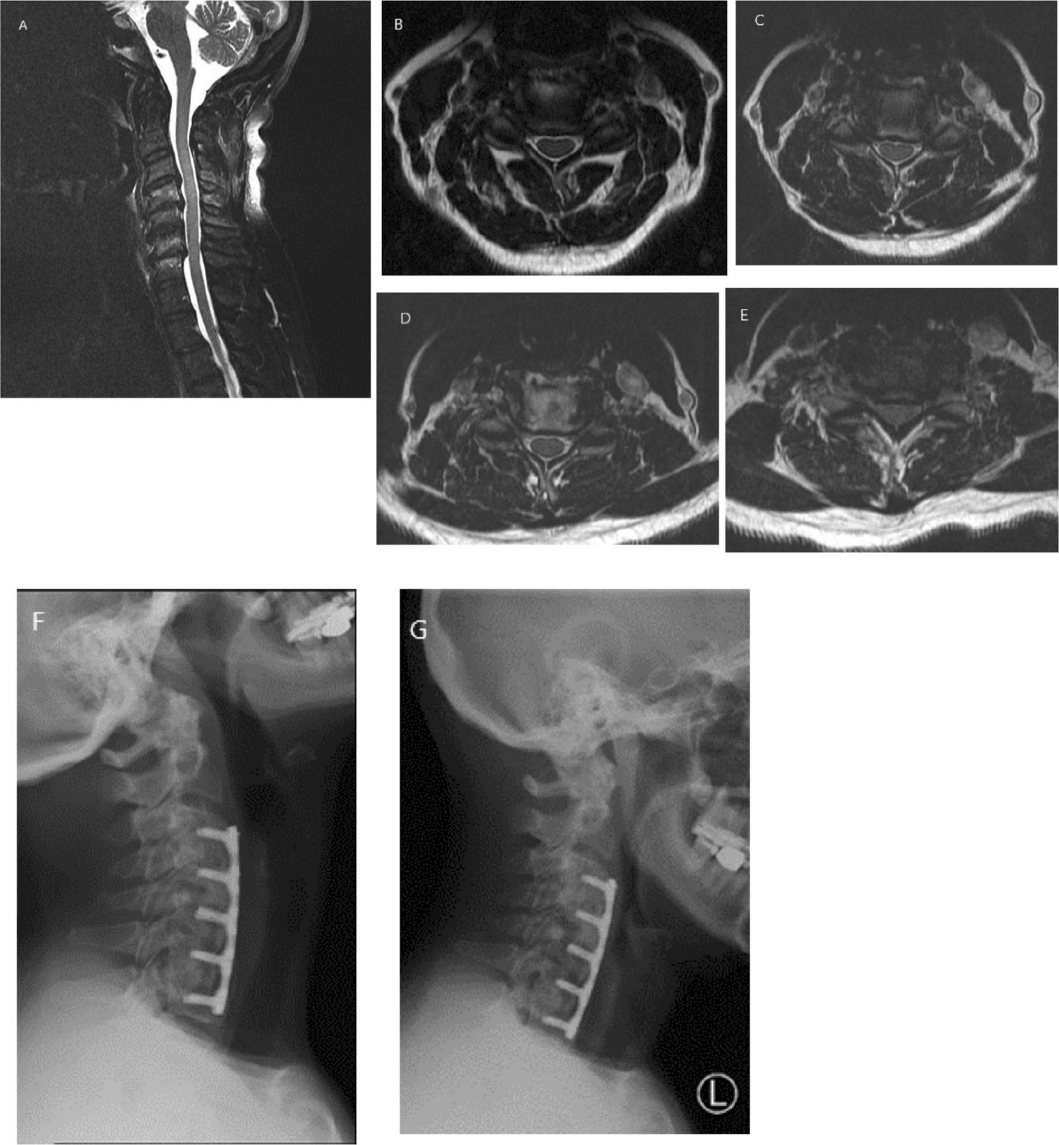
A 57-year-old female with longstanding history of neck pain. Over the past few years, she has also started to notice a gradual decrease in dexterity and numbness in her hands. T2-weighted sagittal and axial magnetic resonance imaging (MRI) scans (A–E) showed multilevel cervical stenosis at the interspace levels C3–C7. The patient underwent a four-level ACDF at the levels C3–C7 with excellent clinical outcome. At the last follow-up, lateral flexion/extension X-rays of the cervical spine (F,G) showed good alignment and solid fusion across each disc segment.
